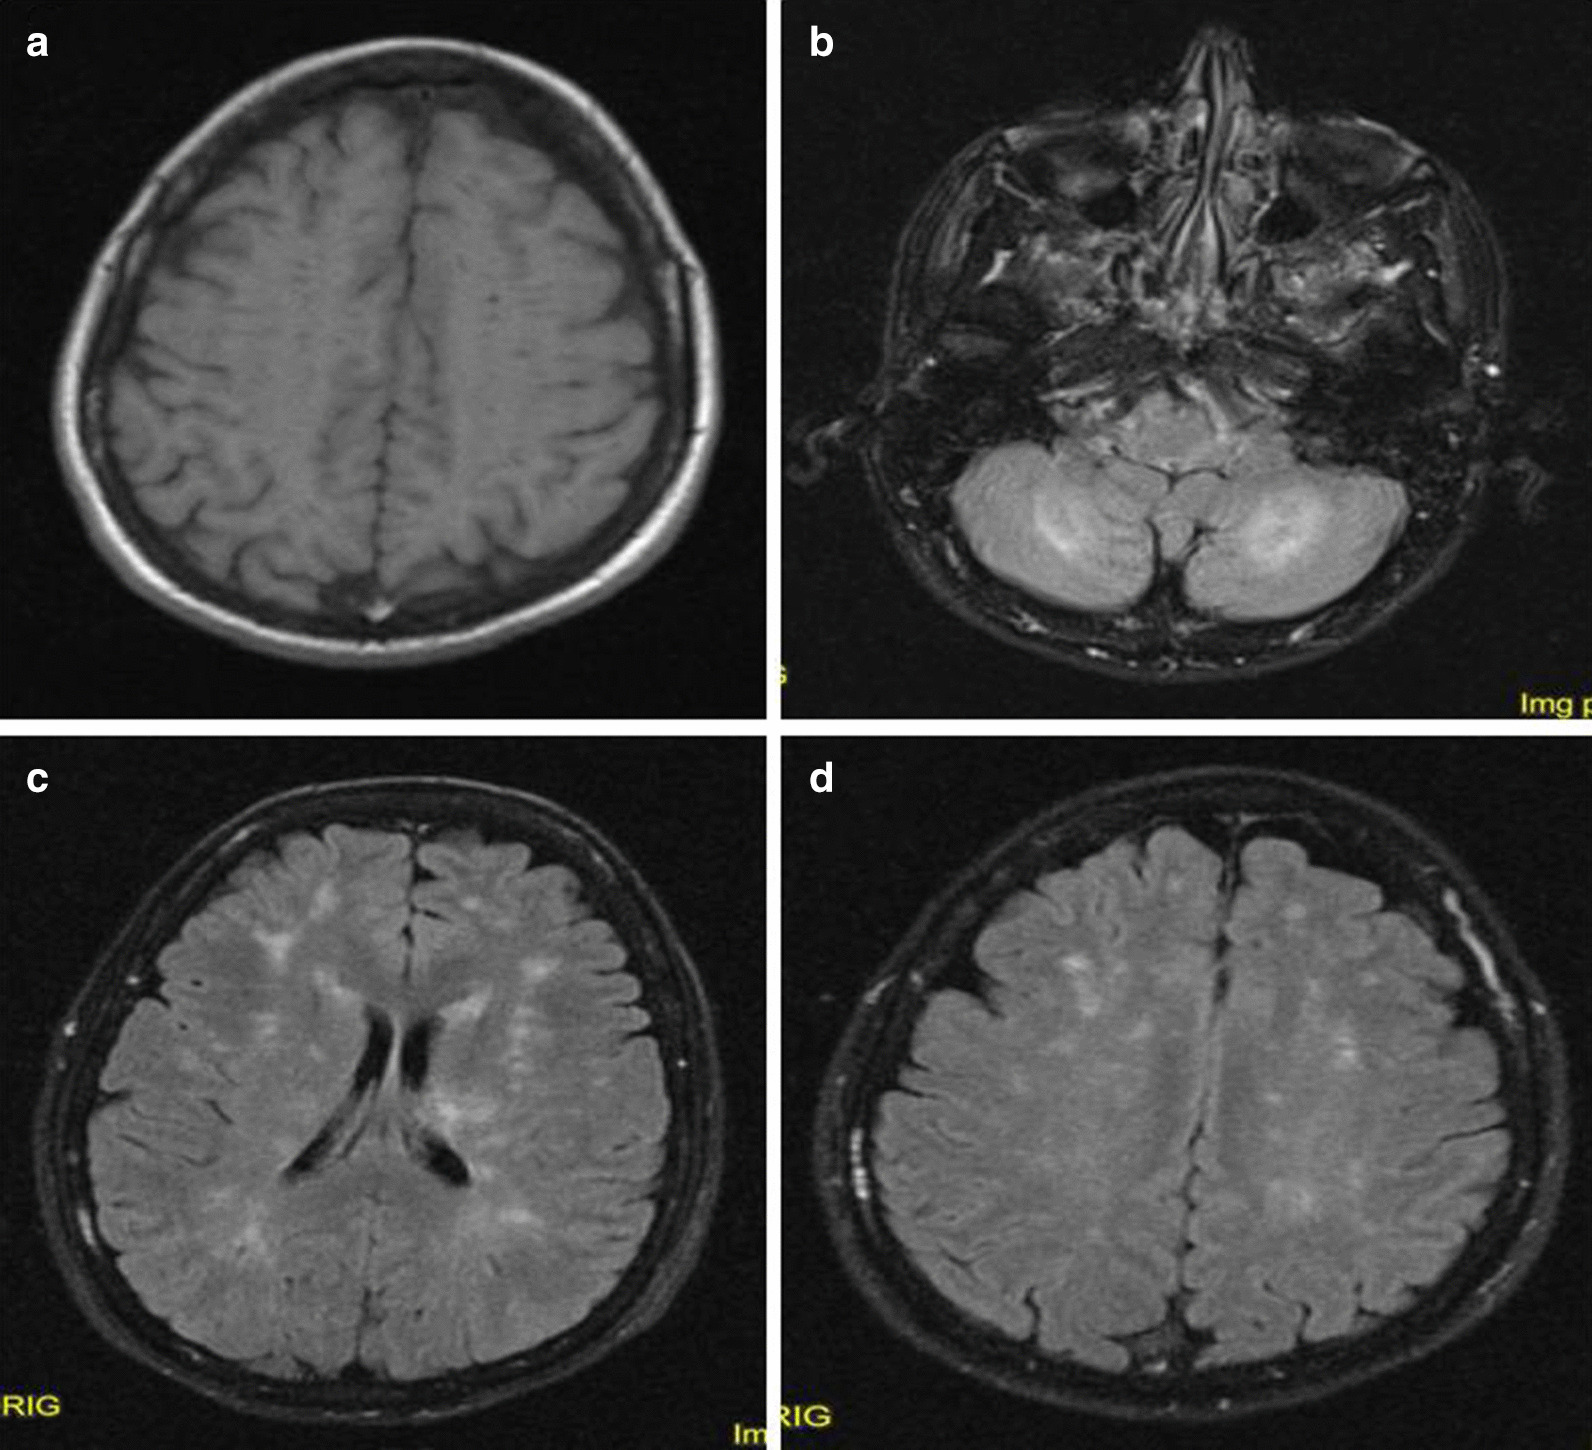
Images from the control MRI scan. a T1-weighted image without meningeal post-contrast enhancement. b FLAIR image demonstrating a reduction of hyperintense lesions in both cerebellar hemispheres. c, d FLAIR images still showing a significant bulk of hyperintense lesions involving periventricular, deep, and juxtacortical white matter
